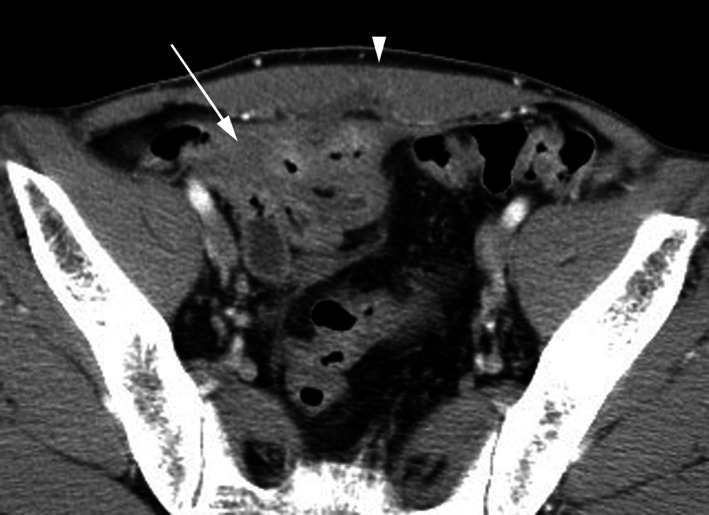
A computed tomography scan revealed the enterocutaneous fistula (arrowhead) and the configuration of mildly dilated bowel loops (arrow).
